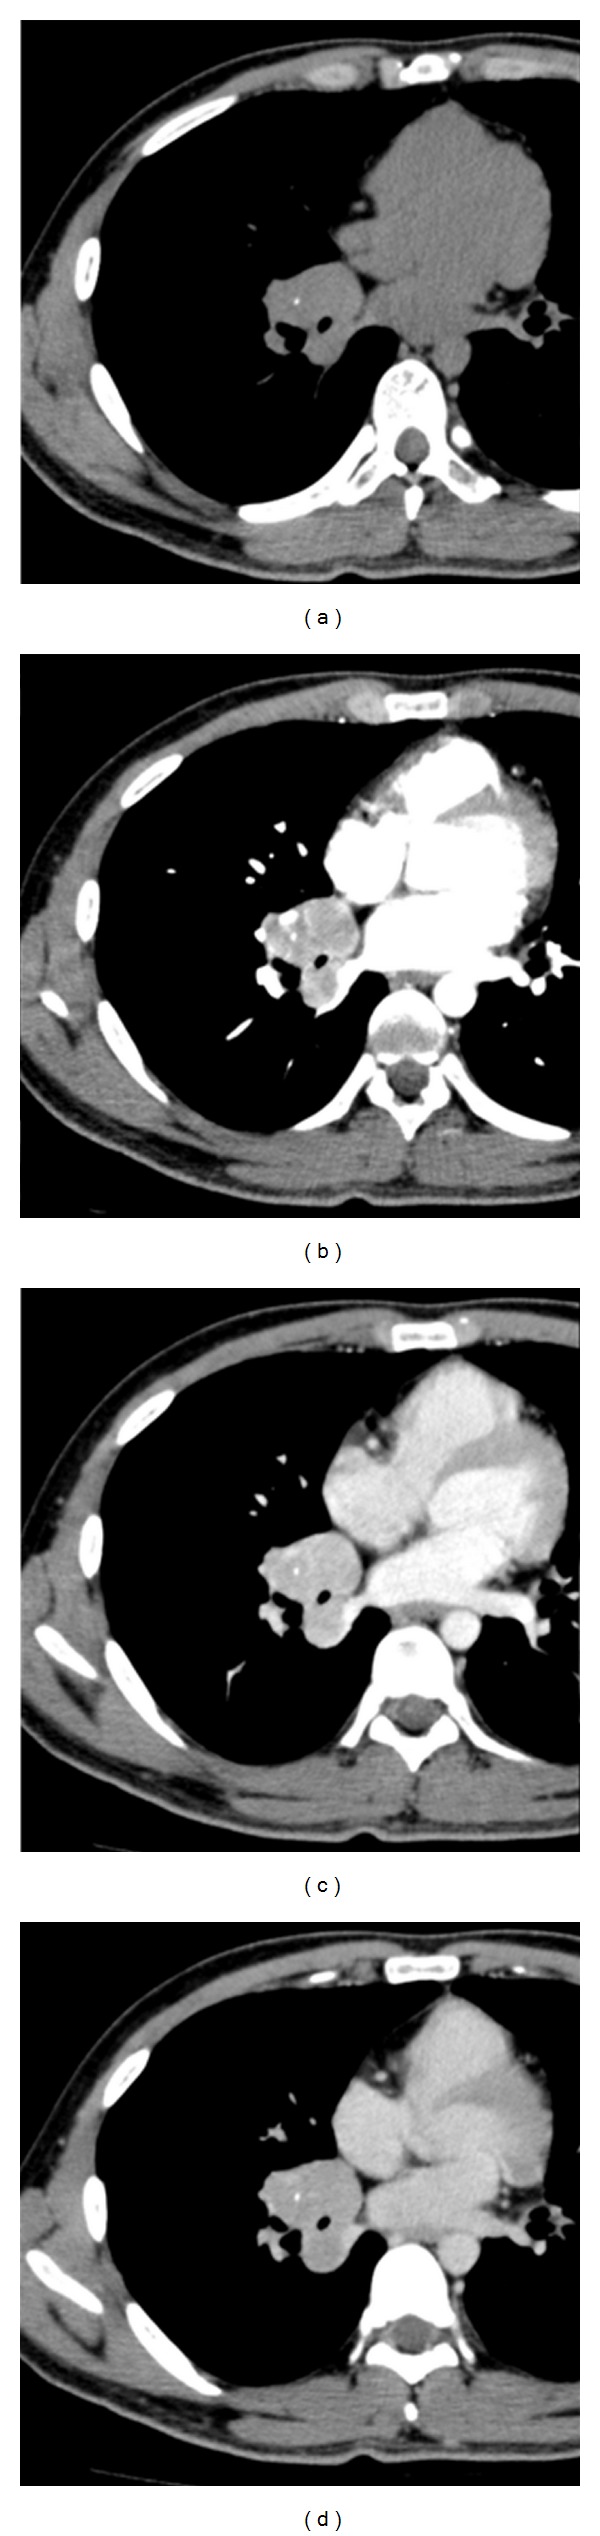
Dynamic CT scans of the chest, in plane (a), arterial (b), portal in-flow (c), and equilibrium (d), show a well-defined, lobulated, soft-tissue mass with small calcifications in the right lower lobe. The periphery of the mass was strongly enhanced in the arterial phase, and the parenchyma of the mass was heterogeneously enhanced in the portal in-flow to equilibrium phase.
